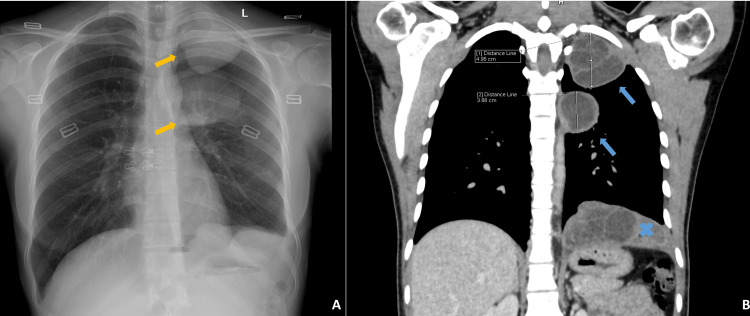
Preoperative imaging findings (A) Chest radiograph showing two well-defined, rounded masses in the upper and middle zones of the left hemithorax (yellow arrows). (B) Contrast-enhanced CT chest (coronal section) demonstrating two well-defined, multilocular, pleural-based, cystic lesions with internal septations (blue arrows), corresponding to the radiographic findings. A multiloculated cystic lesion is also noted in the splenic bed (blue cross).
